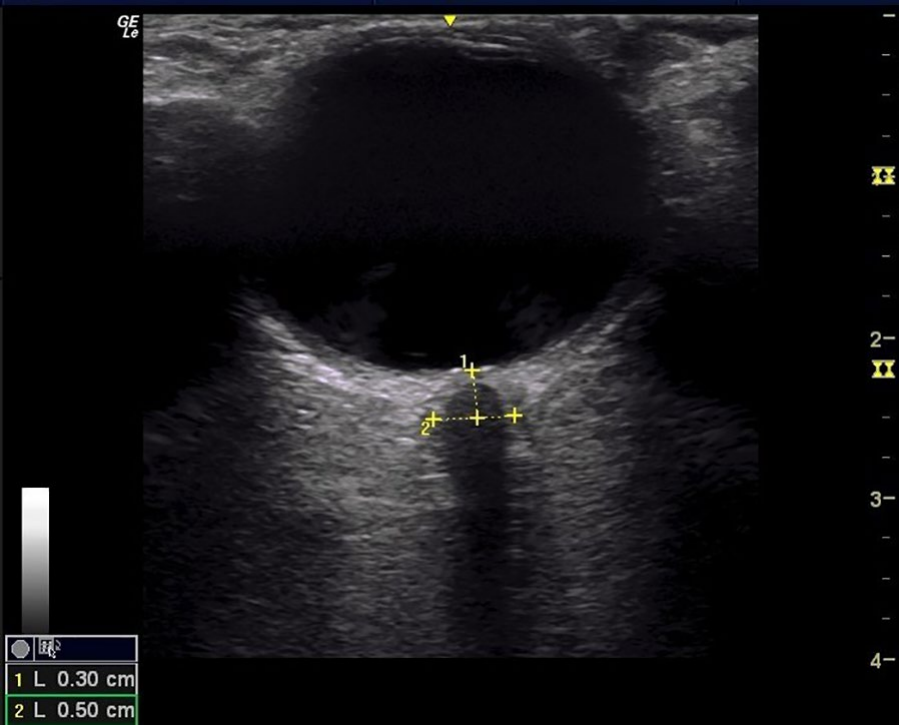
ONSD ultrasound. The ONSD is measured 3 mm behind the retina, from which point a transverse line is drawn from the inner edge to the inner edge of both vertical hypoechoic lines (inner edge of the dura mater)
